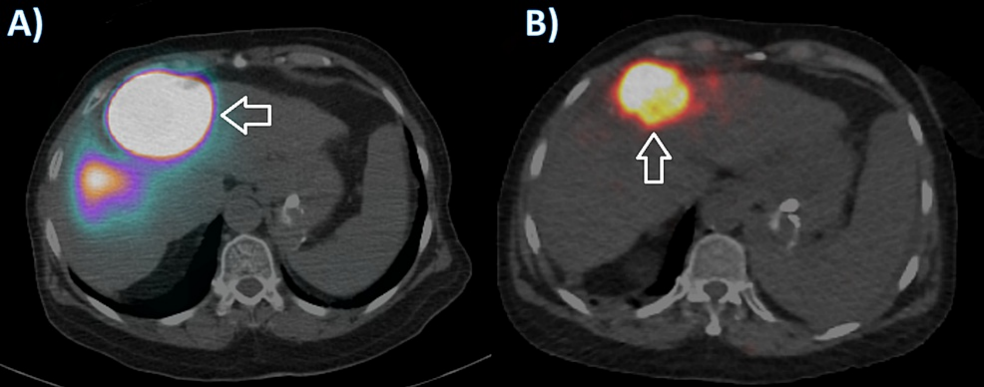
A: axial SPECT-CT image post technetium-99 macroaggregated albumin (Tc-99 MAA) injection confirming radiotracer localization within the known segment IV HCC. B: axial PET scan image following yttrium-90 (Y-90) infusion showing focal intense tracer localization in the known segment IV HCC. SPECT-CT, single-photon emission computed tomography-CT; HCC, hepatocellular carcinoma; PET, positron emission tomography
